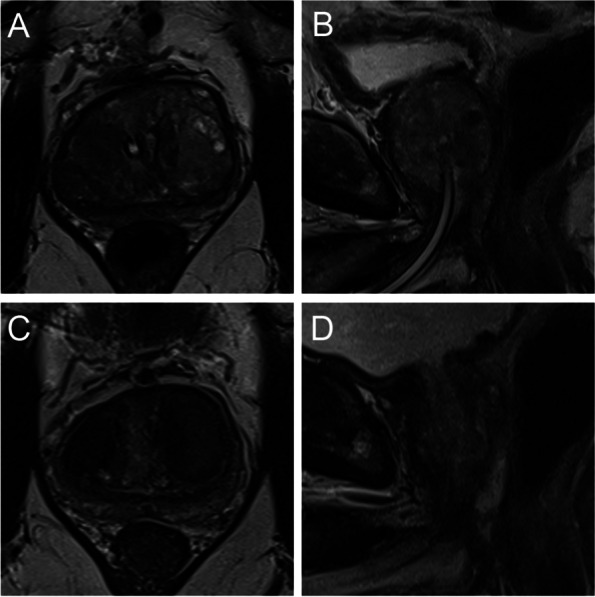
Wasserman type 3, modified BPH classification 3 (biurethral); A T2-weighted axial baseline B T2-weighted sagittal baseline; preurethral dominant hyperplasia without pedunculation; C T2-weighted axial follow-up D T2-weighted sagittal follow-up. Volume reduction of 76 ml to 36 ml (53%); IPSS 24 to 10 (58%)
